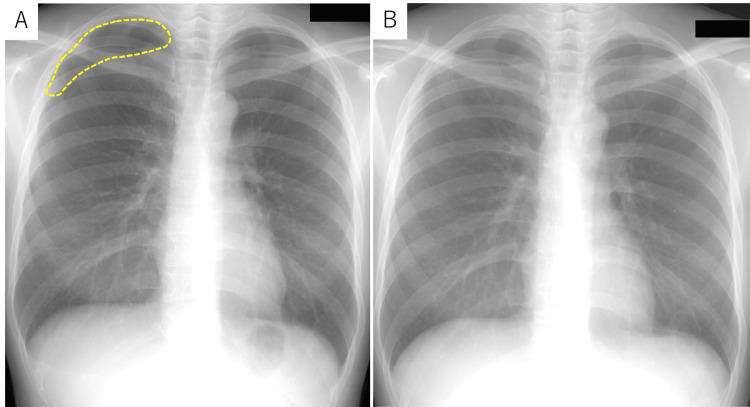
(A) A small pneumothorax is noted in the right hemithorax, primarily involving the right upper lobe (demarcated by a yellow dotted line). (B) Complete resolution of the right pneumothorax is evident on the follow-up chest X-ray obtained eight days later.
